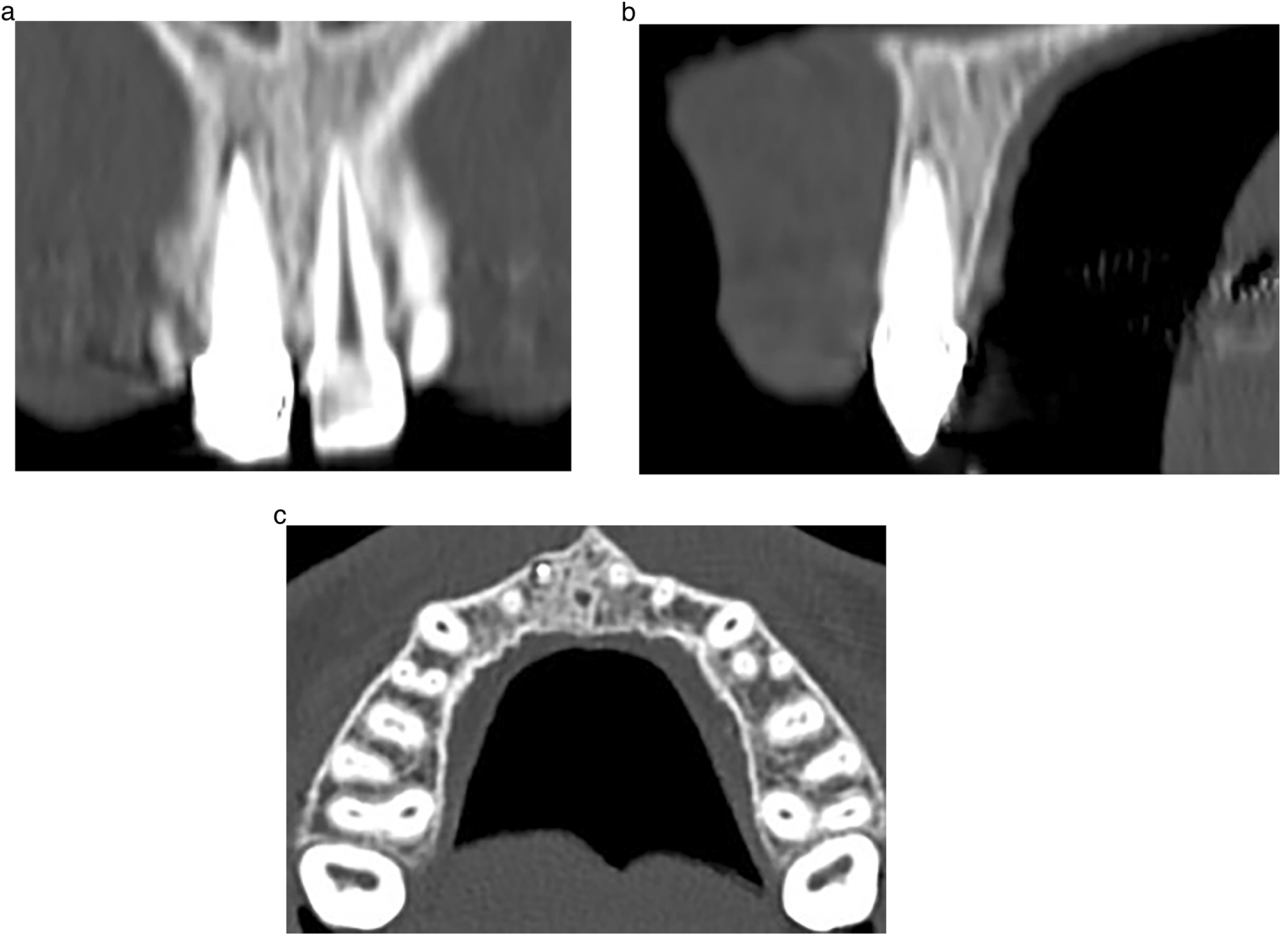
The first radiographical follow- up views. CBCT image after 6 months of treatment; a: Coronal view, b: Sagittal view, and c: Axial view.
